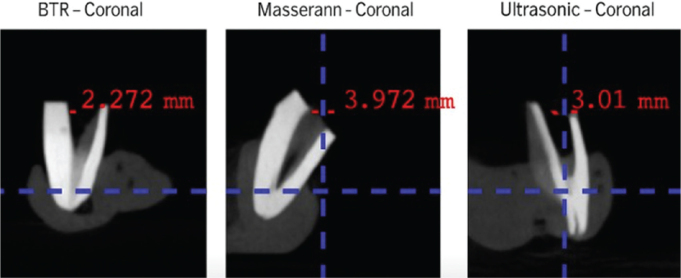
CBCT cross-sectional images of root canals after fractured instrument removal using three different techniques.
